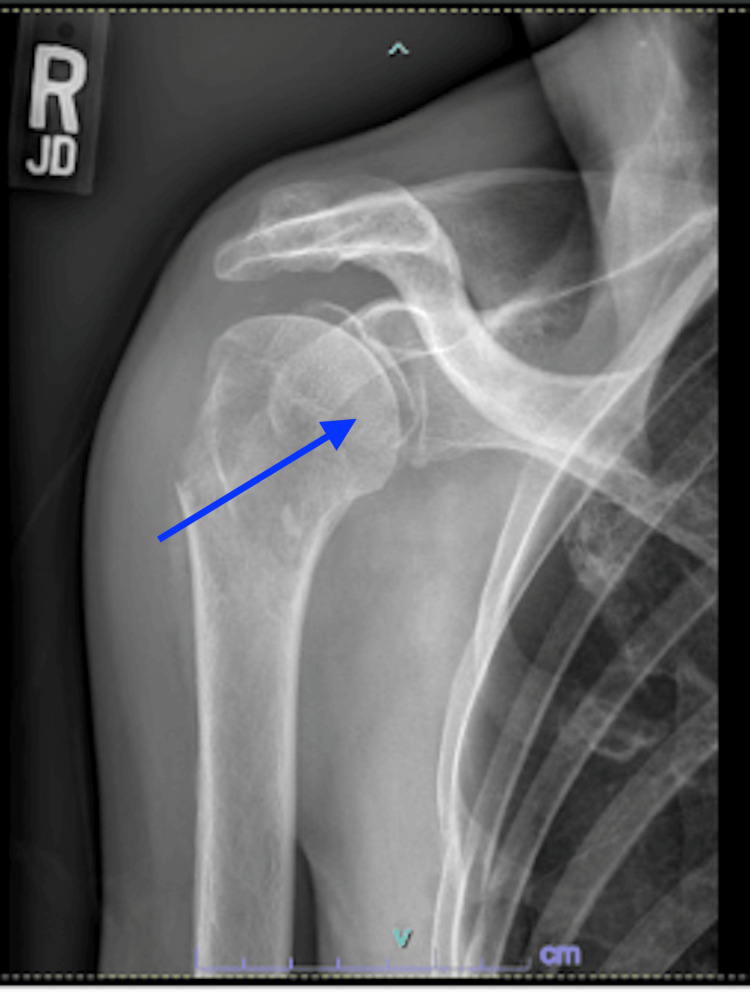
X-ray of the right shoulder on 04/13/2025, showing posterior dislocation of the humeral head and displaced glenoid fracture. This image was taken prior to closed reduction under anesthesia.
